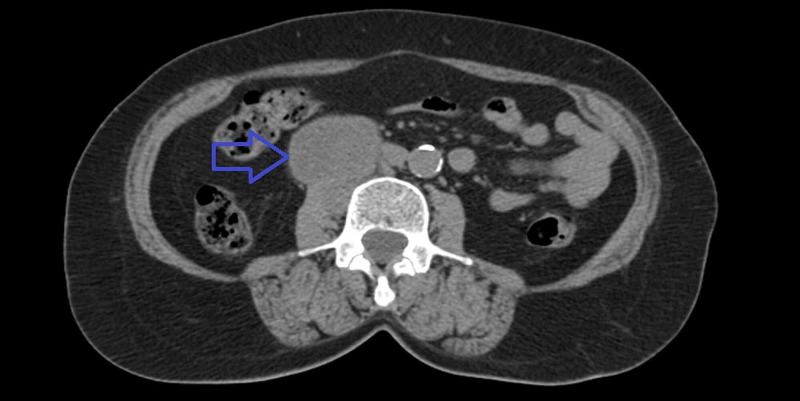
Axial abdominal CT scan showing a right retroperitoneal mass (blue arrow).
